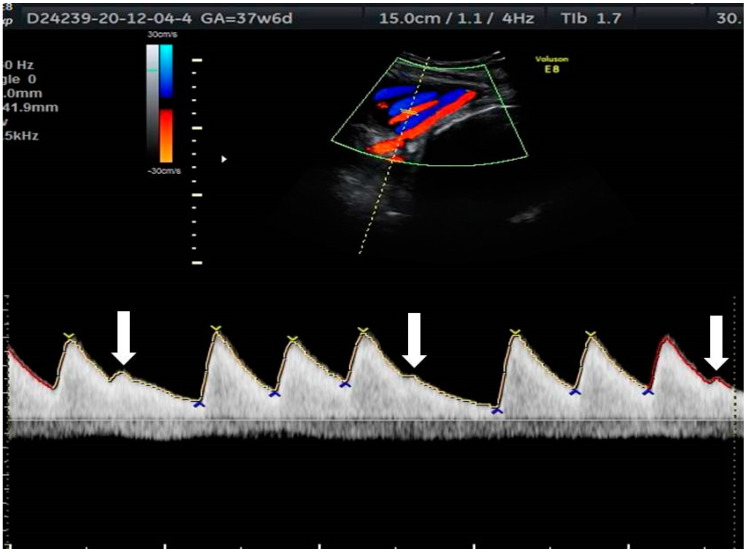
Atrial ectopic beats (indicated by arrows) on the umbilical artery Doppler waveform.
